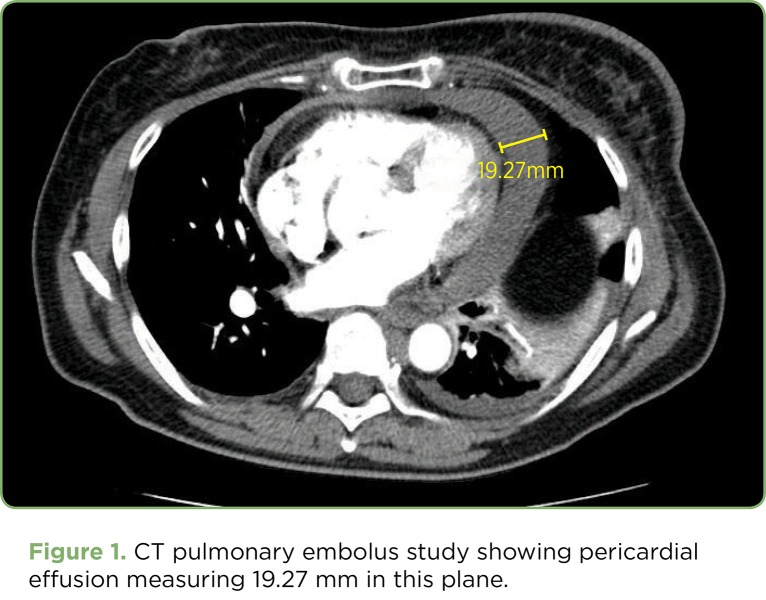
CT pulmonary embolus study showing pericardial effusion measuring 19.27 mm in this plane.
